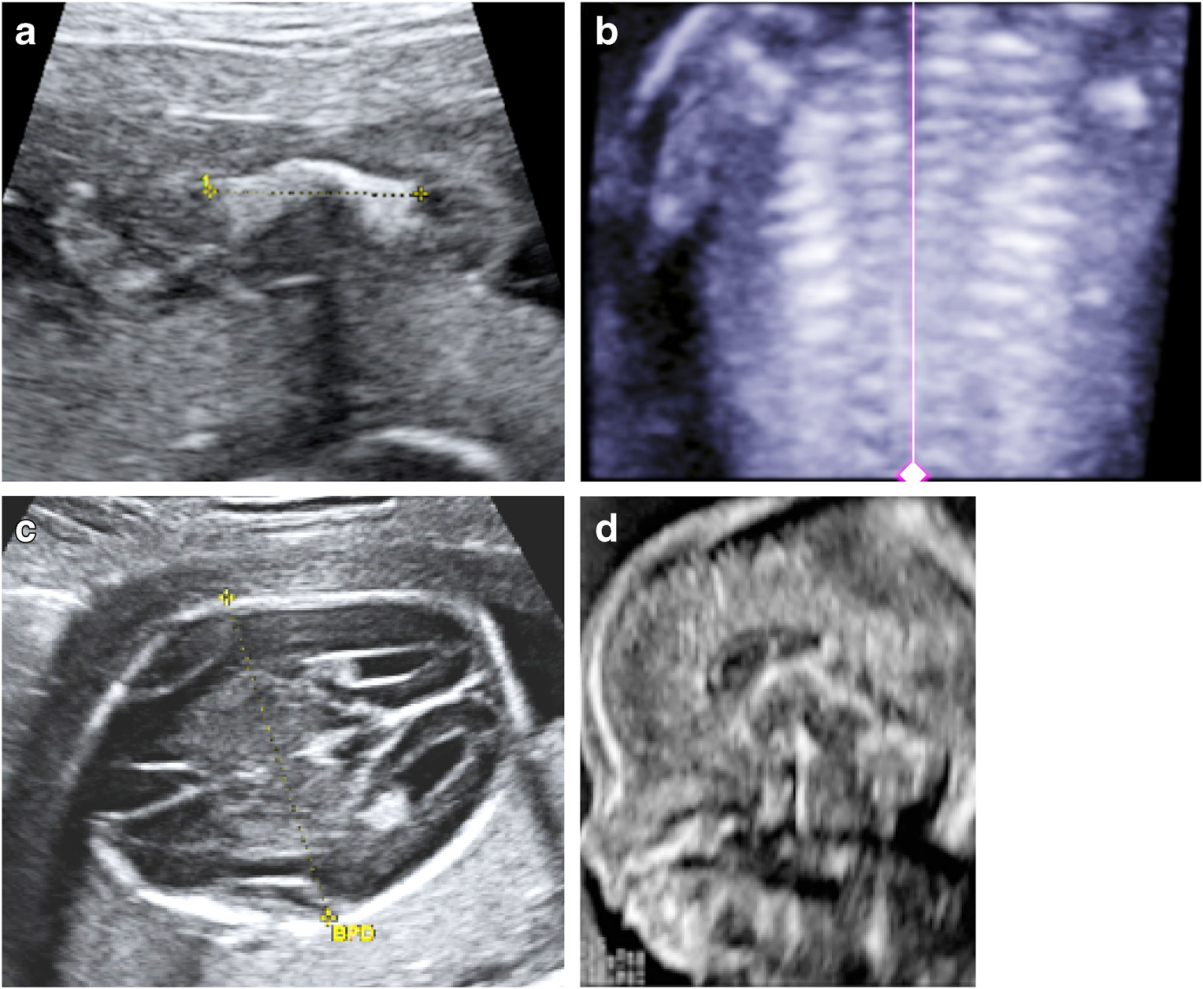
Imaging features of hypophosphatasia of a fetus of unknown gender at 18 weeks’ gestation*.*a-d Prenatal US scan in a longitudinal view of the femur (a) shows a short and angulated femur and a coronal view of the thorax (b) shows short irregular ribs. The vertical line highlights the original software calipers used by the sonographer to document thoracic height. Axial (c) and sagittal (d) views of the skull show a severely underossified cranium. Calipers on the axial view are measuring the biparietal diameter. [Images reproduced with permission from Radcliffe Publishing [38], page 370, case 1, images 1a, 1c, 1f, and 1g]
